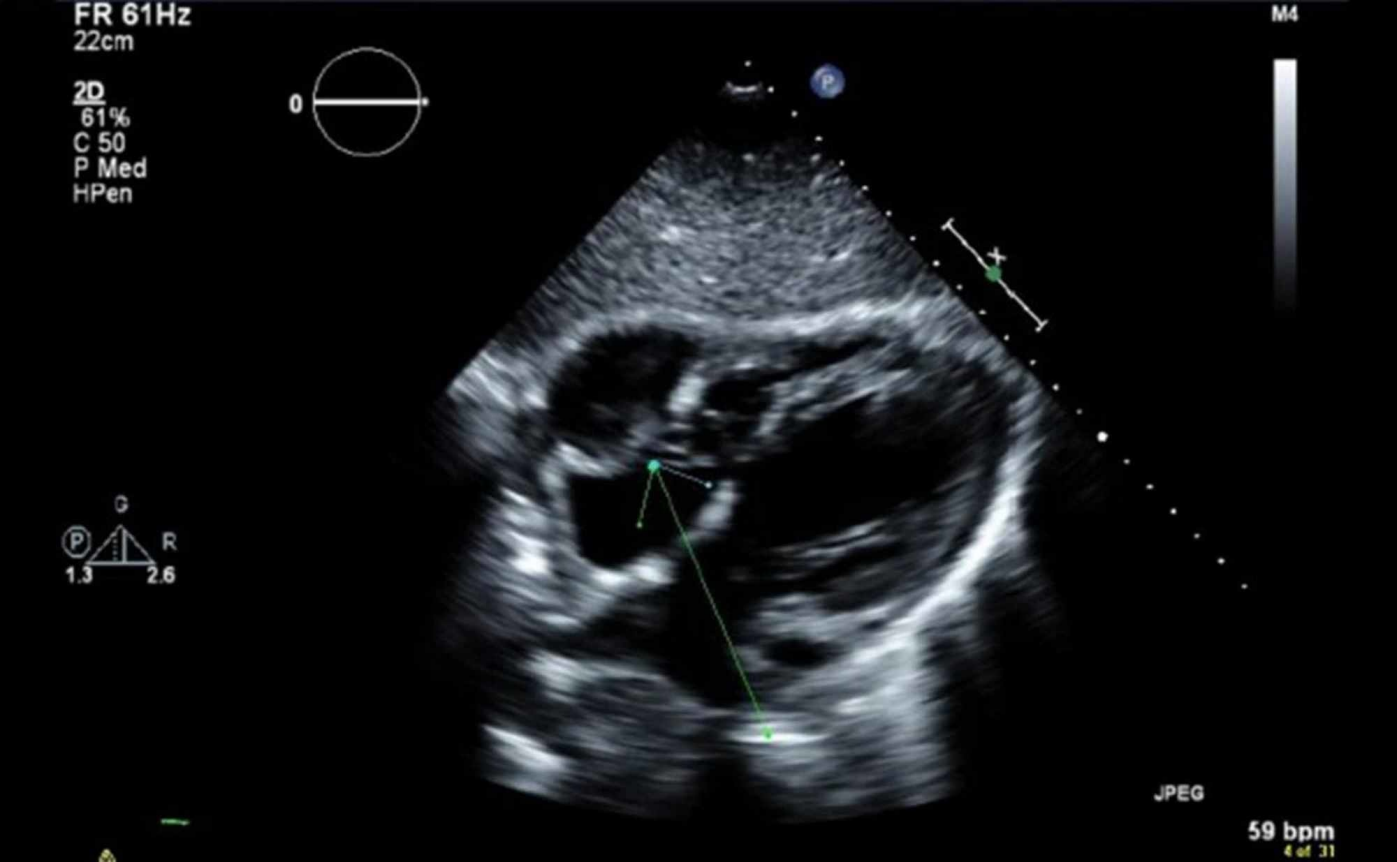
Echocardiogram Mass (arrow) inside the right atrium
